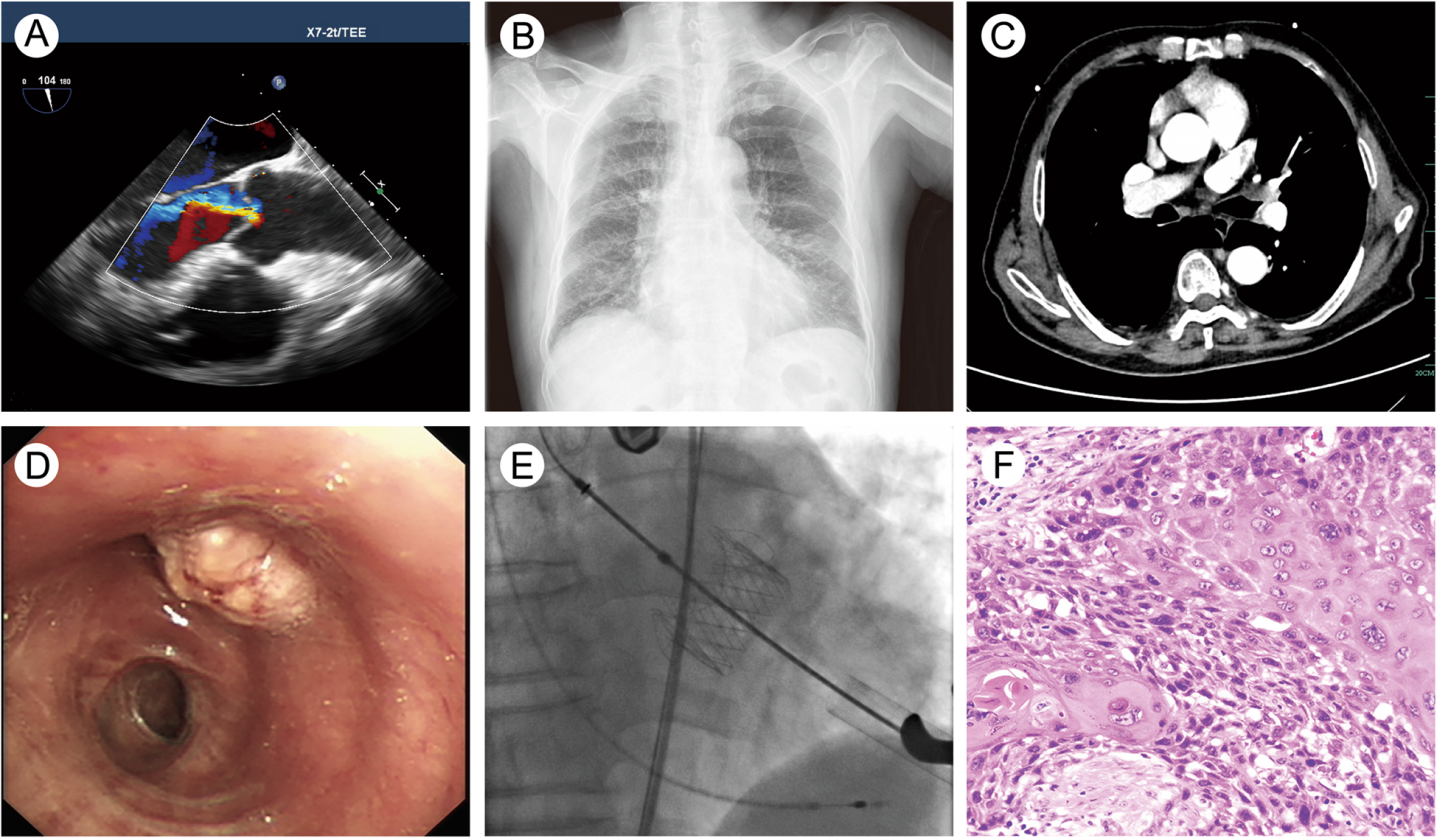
a Transesophageal echocardiography showed that the aortic valve was trilobal, with poor valve closure and moderate to severe regurgitation (Vmax 4.3 m/s, LVDD 62 mm, LVEF 55%). b The chest X-ray indicated chronic bronchitis, emphysema and two lung infections. (C) The CT scan showed a partial blockage in the left upper lobe bronchus. d The bronchoscope showed a tumour in the left upper lobe. The distance from the carina is more than 2 cm, and a later biopsy revealed squamous cell carcinoma. e The intraoperative angiography showed a 29 J-Valve was implanted from the apex of the heart. f The pathological findings revealed central highly differentiated squamous cell carcinoma (HE × 400)
